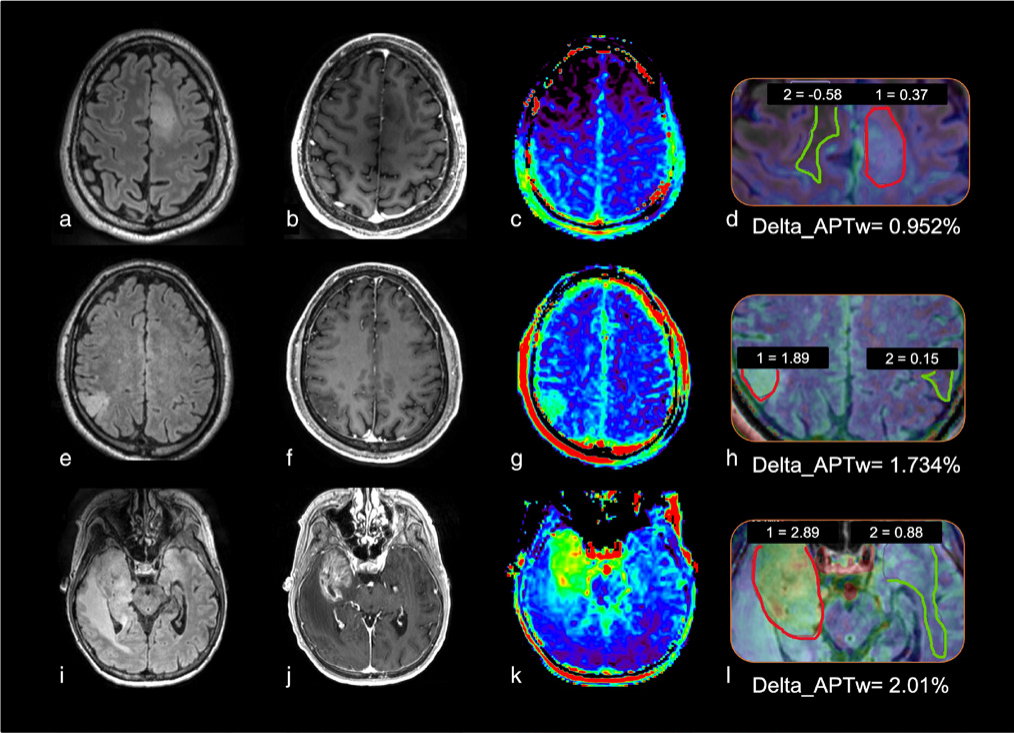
Example of how APTw signal intensity increases as a function of tumor grade *(case courtesy of Sotirios Bisdas and Laura Mancini, University College London)*. (a–d) Oligodendroglioma, IDH-mutant and 1p/19q codeleted, WHO grade 2. (e–h) Oligodendroglioma, IDH-mutant and 1p/19q codeleted, WHO grade 3. (i–l) glioblastoma, IDH-wildtype, WHO grade 4. Lesion measurements analysis is displayed in D, H and L: average values of APTw metrics are computed after delineation of a region of interest (ROI) in the tumor lesion and in the contralateral normal-appearing white matter (cNAWM). Delta_APTw is calculated after the subtraction of the APTw ROI lesion values with the cNAWM ROI values. (a, e, l) FLAIR sequence. (b, f, j) T1w after injection. (c, g, k) APTw maps. (d, h, l) zoom on APTw maps superposed with FLAIR images and Delta_APTw measurements. APTw, amide proton transfer-weighted; cNAWM, contralateral normal-appearing white matter; FLAIR, fluid attenuated inversion recovery; ROI, region of interest.
